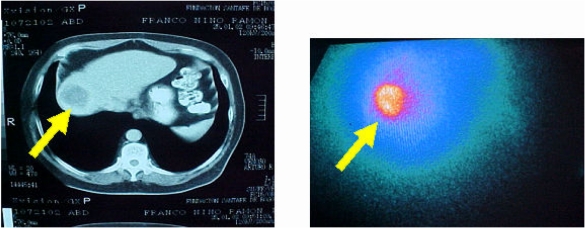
CT scan and Rhenium-188 Lipiodol treatment scan in a patient with HCC.
